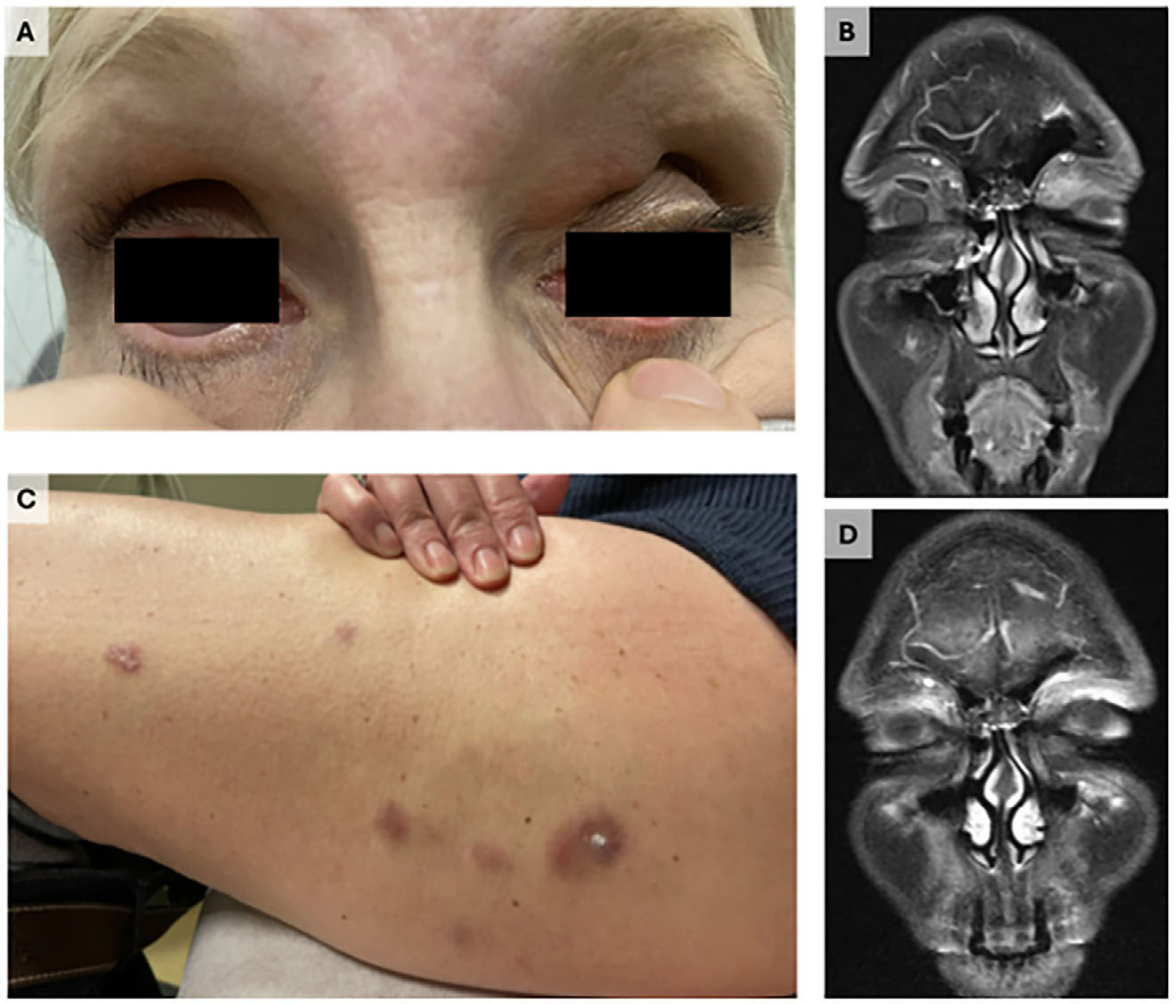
Physical examination demonstrated necrobiotic xanthogranuloma skin lesions of the patient's left eyelid (A) and left upper thigh (C). MRI of the orbits demonstrated a left superomedial preseptal mass (B) that resolved after treatment with plasma cell‐directed therapy (D).
